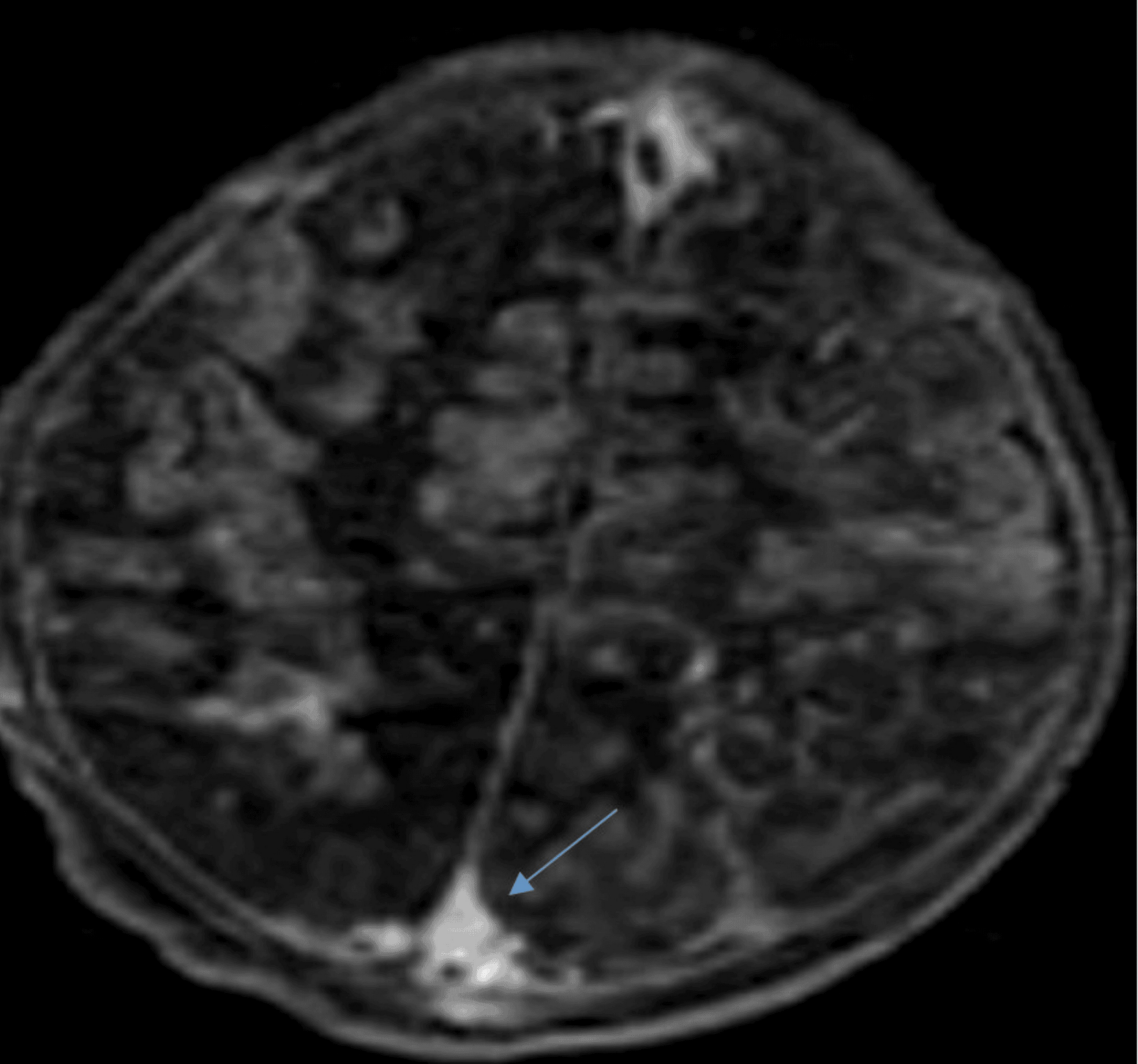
Brain MRI in axial T1 sequence with gadolinium injection showing thrombosis of the superior sagittal sinus (blue arrow) MRI: magnetic resonance imaging
